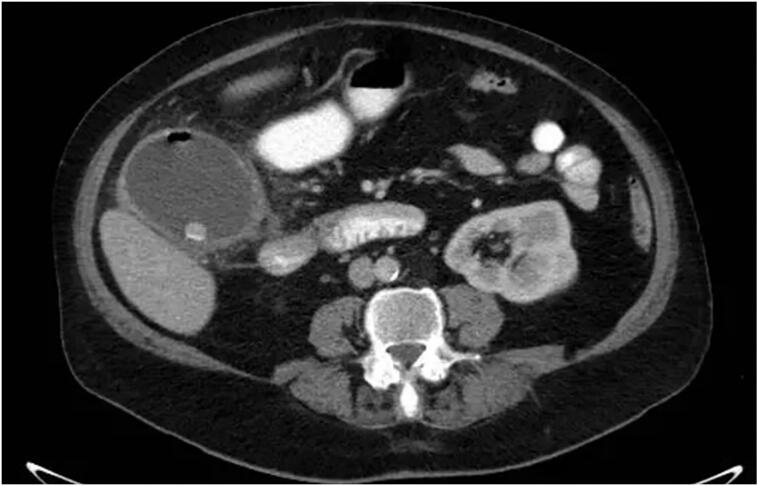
Axial CECT (contrast-enhanced computed tomography) of the abdomen: Enlargement and thickening of the gallbladder wall are evident. The presence of numerous stones, along with a minor quantity of air within the wall and lumen, and pericholecystic fat stranding were observed. These observations align with a diagnosis of emphysematous cholecystitis.
